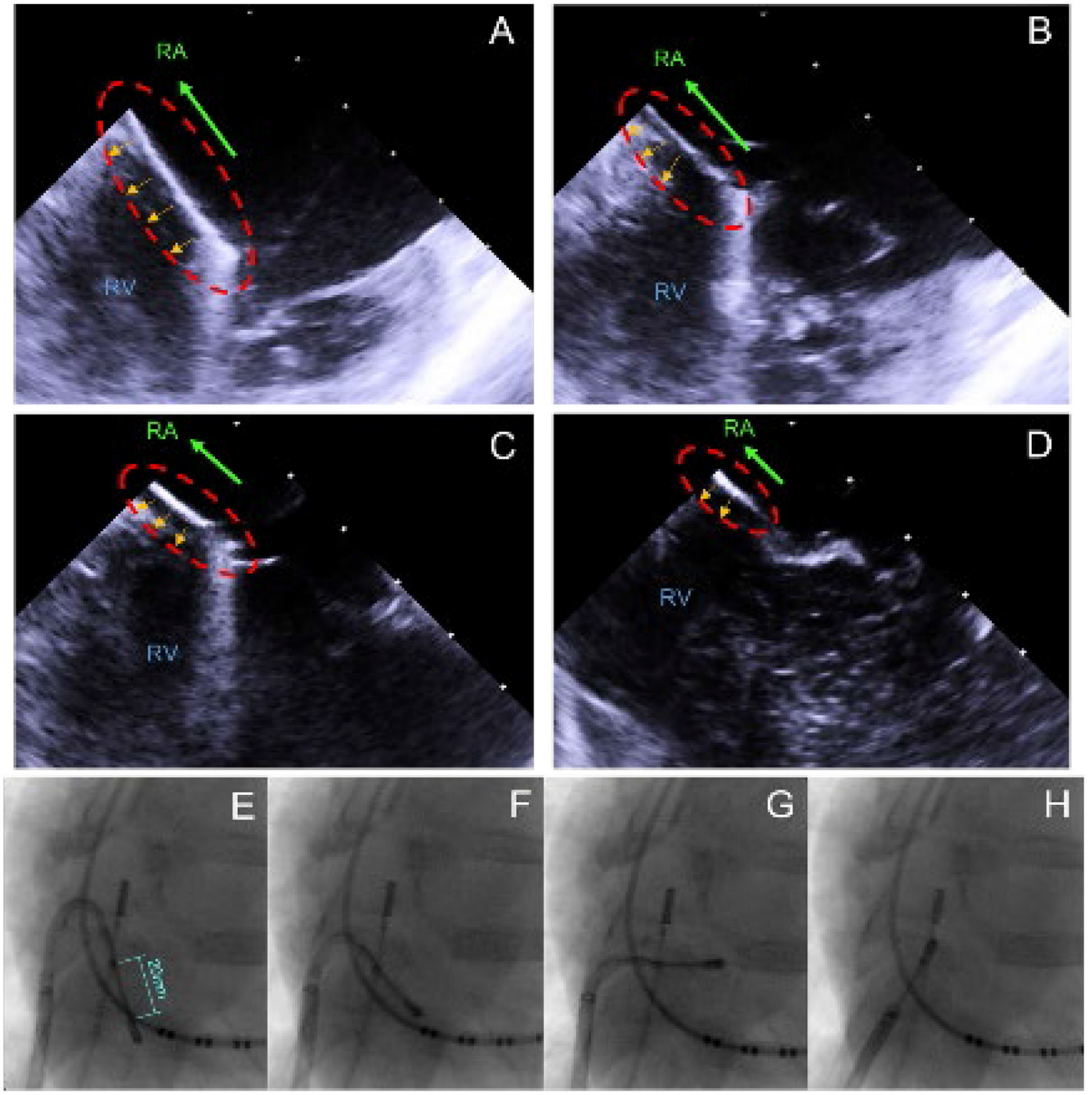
ICE/X-ray recordings captured during the CTI ablation, visualizing the step-wise retraction from the RV/TCV toward the IVC. (A) Initial position with the catheter partially in the RV and on the TCV. (B–D) Stepwise retraction into the IVC. (E) Initial position with the catheter partially in the RV and on the TCV. (F–H) Stepwise retraction into the RA.
